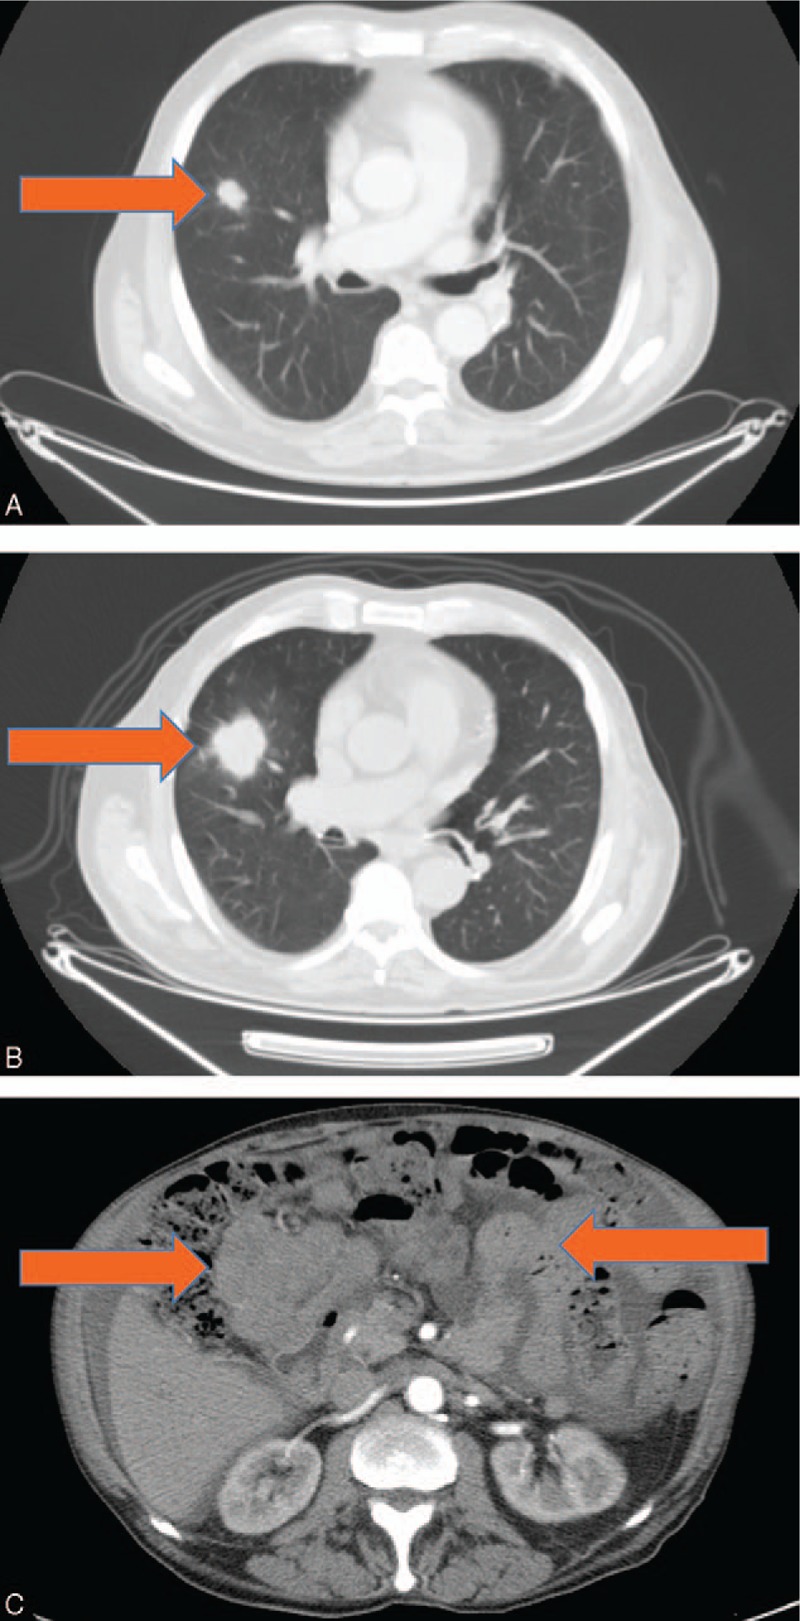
Relevant figures from CT-scans collected throughout the patient's clinical history. The arrows indicate lesions of interest. Notably, as the patient could not provide CT-scans performed before April 2009 in a different Institution, pictures of the lung adenocarcinoma located in the left lower lobe are not available. (A) CT-scan picture showing the SCC located in the right upper lobe in September 2010, before being treated with carboplatin-based chemotherapy; (B) CT-scan picture showing the same tumor (SCC) as in November 2011, progressing after carboplatin-based chemotherapy and periodical follow-up; (C) CT-scan picture showing diffuse abdominal lesions of PM.
